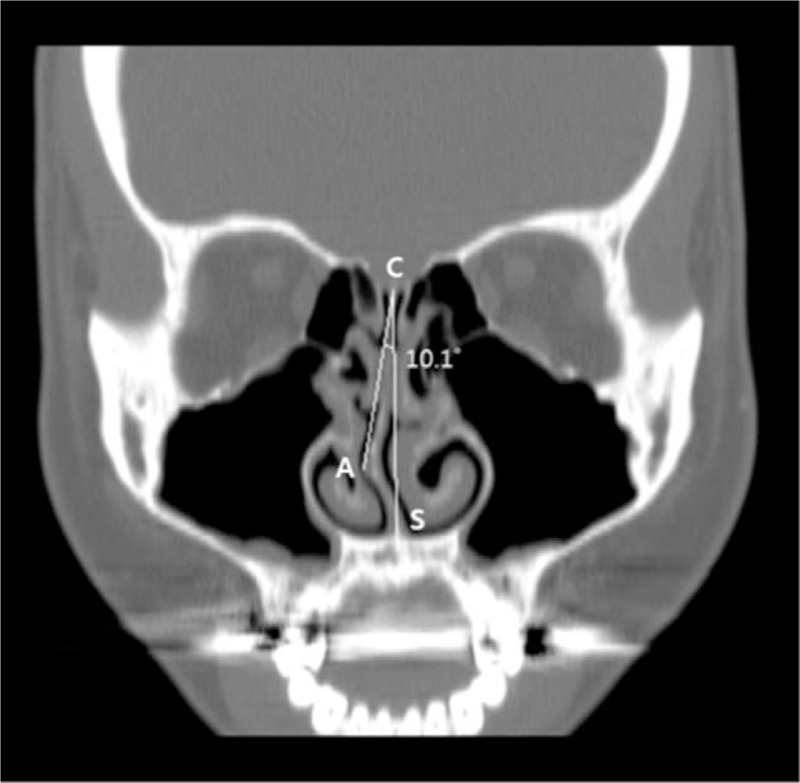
Assessment of septal deviation with computed tomography (CT). The presence of septal deviation was evaluated using coronal CT scans. The superior insertion of the nasal septum at the crista galli (C), its inferior insertion at the level of the anterior nasal spine (S), and the apex of the nasal septal deviation (A) were all marked on the CT scan. The septal deviation angle was defined as the angle between the midline (C–S line) and the line from the crista gali to most markedly deviated point (C–A line). CT = computed tomography.
